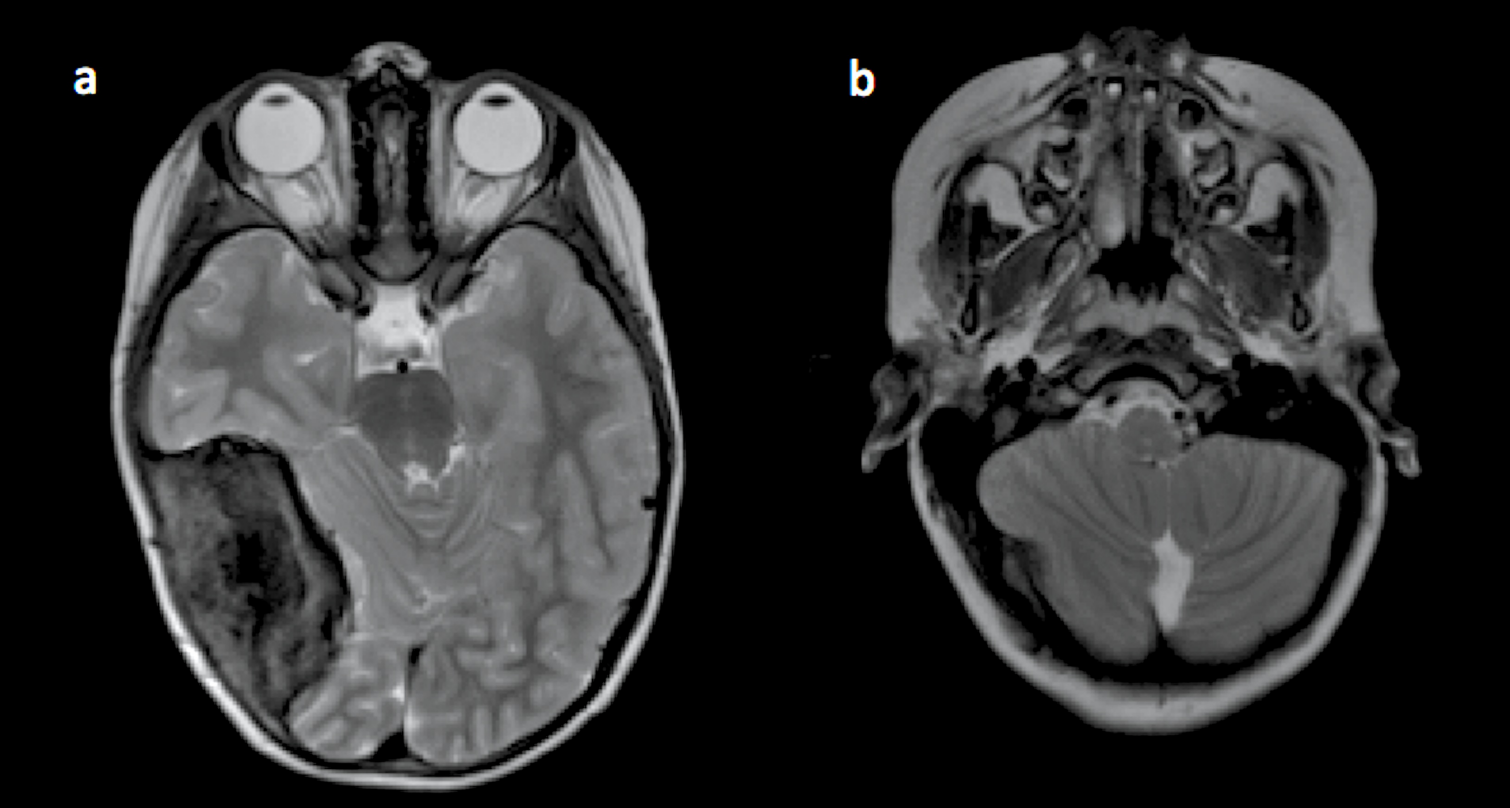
Presenting magnetic resonance imaging (MRI) prior to cavernoma formation (a) T2 axial MRI demonstrating a large R parietal lesion consistent with melanotic primitive neuroectodermal tumor (PNET), which did undergo gross total resection. (b) T2 axial MRI from 2007 demonstrating no lesions in the right medullary region.
